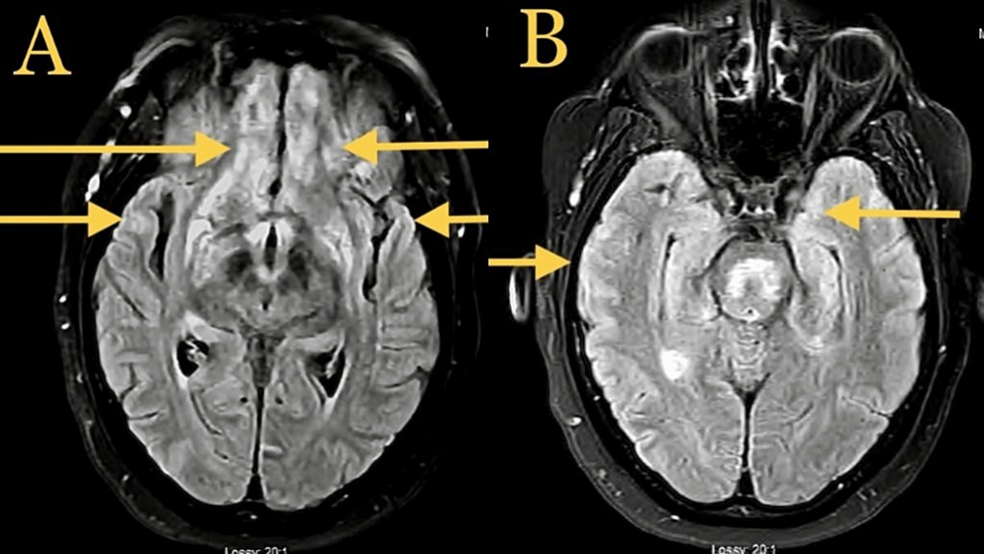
MRI head without IV contrast, axial view in FLAIR SPIR demonstrating diffuse nonspecific signal abnormality in frontal and temporal lobes indicated by yellow arrows
